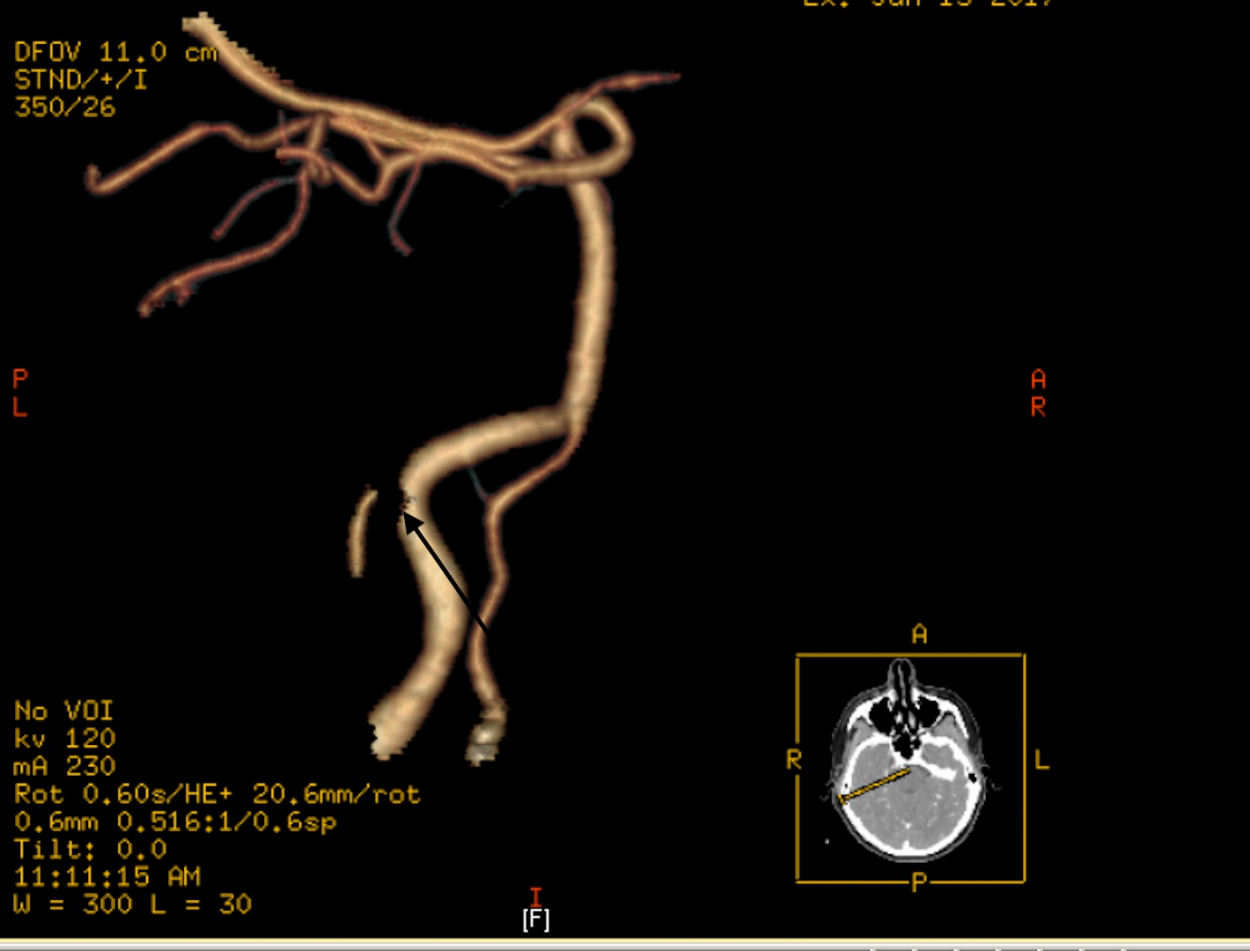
Computed Tomography Angiography (CTA) Neck CTA neck showing dissection of the right internal carotid artery at the C1-C2 level associated with infiltrative changes within the right carotid space
